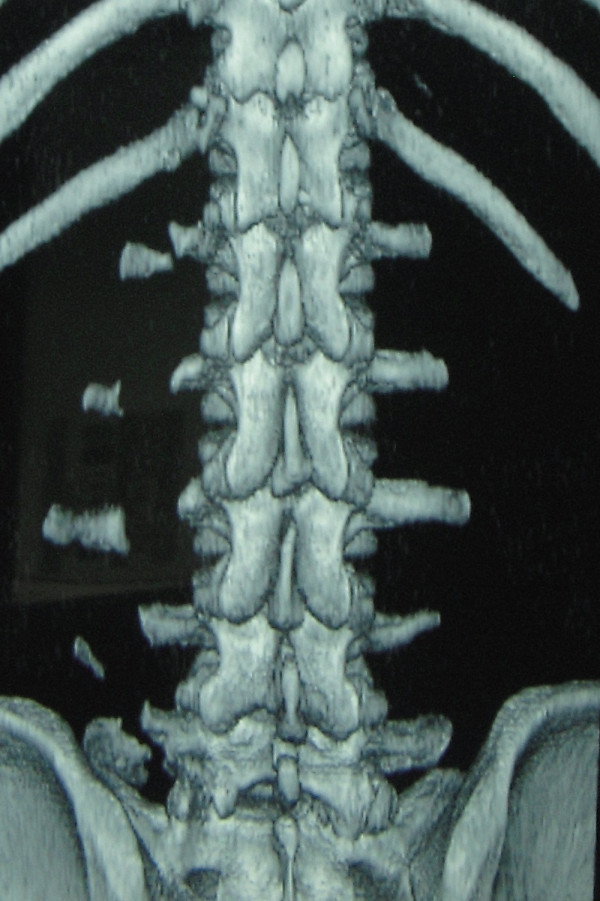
CT scan with 3-D reconstruction image of the same patient; posterior aspect.
